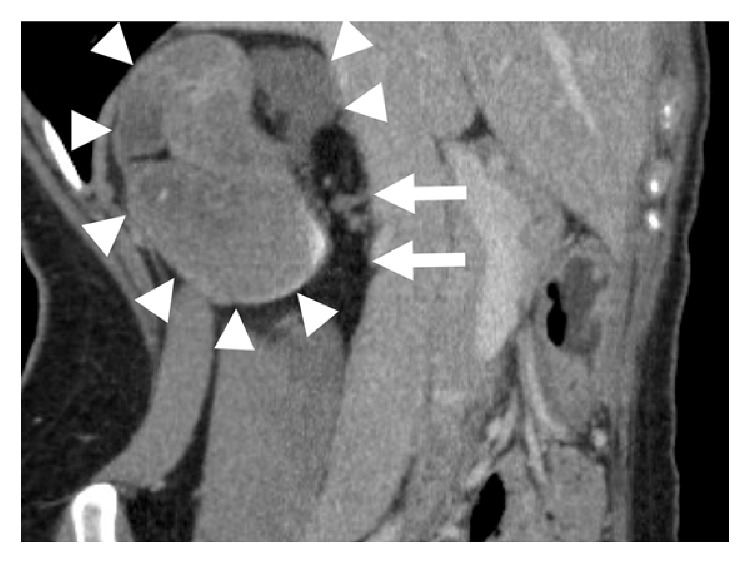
Oblique MPR images of contrast-enhanced CT scan. A tortuous tubular complex shape of the mass (arrowheads) was clearly observed. The nodular fatty component of the mass continued to the normal retroperitoneal fat (arrows).
